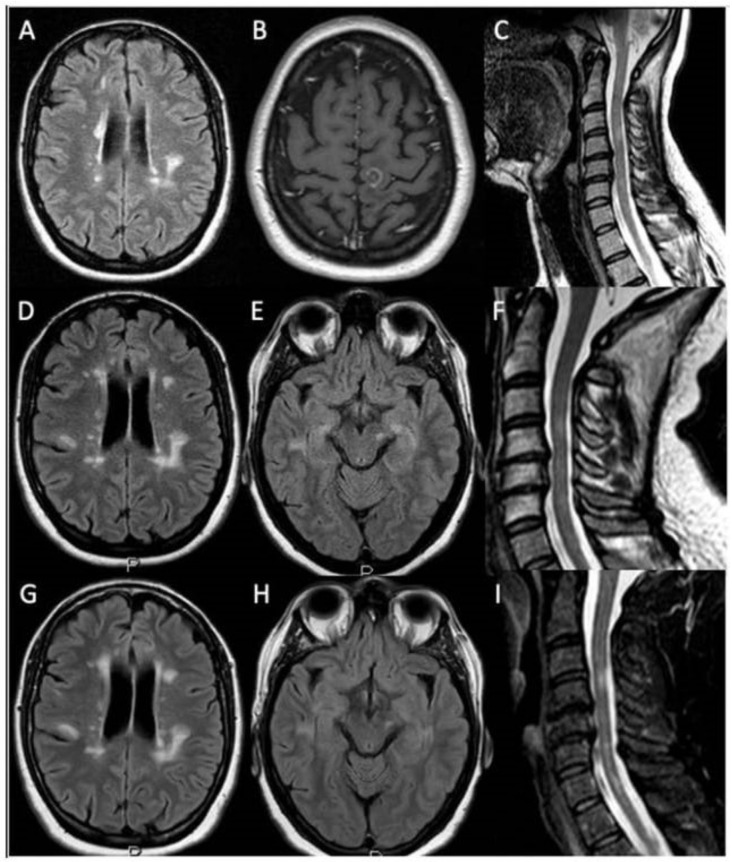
Patient’s brain and spinal cord MRIs. 1.5 tesla brain and spinal cord MRI obtained before and after IV gadolinium injection. November 2006: axial fluid-attenuated inversion recovery (FLAIR) and t2 weighted sequences showed the presence of multiple demyelinating lesions located in the periventricular and hemispheric deep white matter (A) and cervical spinal cord (C), including one contrast enhancing lesion in the left fronto-parietal juxtacortical white matter (B). November 2011: FLAIR and t2 weighted sequences showed the presence of multiple demyelinating lesions within the periventricular and hemispheric deep white matter (D), left mesencephalic (E) and cervical spinal cord regions (F). February 2018: brain MRI FLAIR sequences showed the presence of multiple demyelinating lesions located in the periventricular and hemispheric deep white matter (G) and left mesencephalic region (H) (superimposable on previous MRI studies) without new contrast enhancing lesions. Cervical spinal cord t2 weighted sequences displayed the presence of multiple demyelinating lesions (unchanged with respect to the previous MRI study) together with cervical spondylosis with posterior osteophytosis without spinal cord and nerve roots compression (I).
